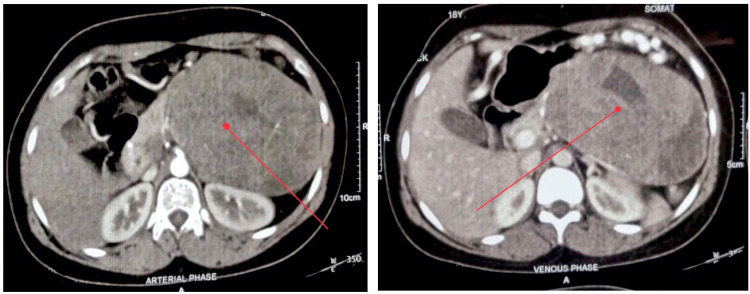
Contrast-enhanced CT abdomen showed well-defined, heterogeneously enhancing, soft tissue density lesion with areas of necrosis arising from the body and tail of the pancreas. The splenic vein was thrombosed with multiple perigastric collaterals.
